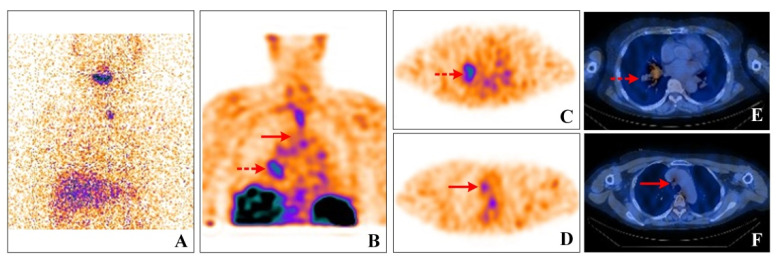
^131^I whole-body scan(WBS) (A), ^99m^Tc-3PRGD2 SPECT/CT WBS (B), ^99m^Tc-3PRGD2 SPECT/CT imaging (C–F). A 57-year-old female with papillary thyroid carcinoma (PTC) was suspected of pulmonary metastasis, as multiple pulmonary nodules and enlarged mediastinal lymph nodes (LNs) were found during the preoperative work-up. The tests on post-surgery found undetectable stimulated thyroglobulin levels. Four weeks post-surgery, 150 mCi radioactive iodine therapy (RAI) was administered. Except for faint uptake within the thyroid bed, no concentration in the pulmonary lesions was found (Figure 1A). Six months after RAI, thoracic CT demonstrated apparent progression of the pulmonary lesions. An irregular mass in right hilar with multiple small nodules was detected. Tuberculosis (TB) or primary lung cancer was suspected. At the same time, all the lab tests, including the T-SPOT, TB sputum smear, TB-DNA, and serum tumor markers (CEA, NSE, and SCC), showed negative results. As integrin αvβ3 receptor imaging has advantages in evaluating hyper vascularization in pulmonary lesions [1,2], our center conducted a clinical trial of RGD imaging concerning thyroid cancer and related malignancies. Given that the traditional imaging features may overlap with other pathologic entities in the lung, an integrin αvβ3 receptor imaging ^99m^Tc-3PRGD2 SPECT/CT was performed. Significant focal uptake of ^99m^Tc-3PRGD2 was found in the hilar mass (Figure 1B, dotted arrows; Figure 1C,E), as well as in the enlarged lymph nodes in the neck and mediastinum regions (Figure 1B, dashed arrows; Figure 1D,F). Primary lung cancer was suspected, but flexible bronchoscope biopsy showed non-caseating epithelioid cell granuloma nodules in the lesion.
